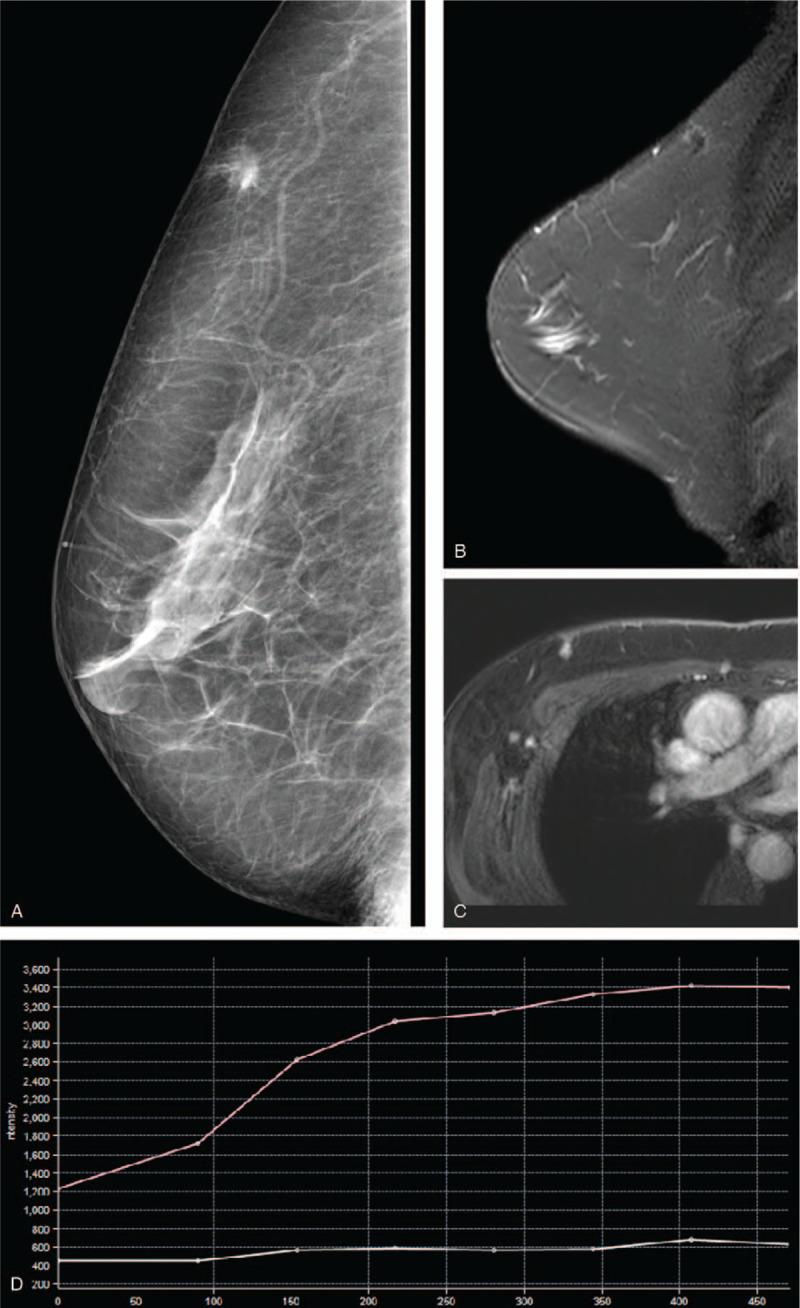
(A) Mediolateral oblique mammogram shows a subcutaneous mass with spiculate boundary and adhesion to the adjacent skin. Magnetic resonance imaging shows an irregular nodule with an isointense signal on T2-weighted sequence image (B), uniformly enhanced after gadolinium injection (C) and I type of TIC curve (D). TIC = time-signal intensity curve.
